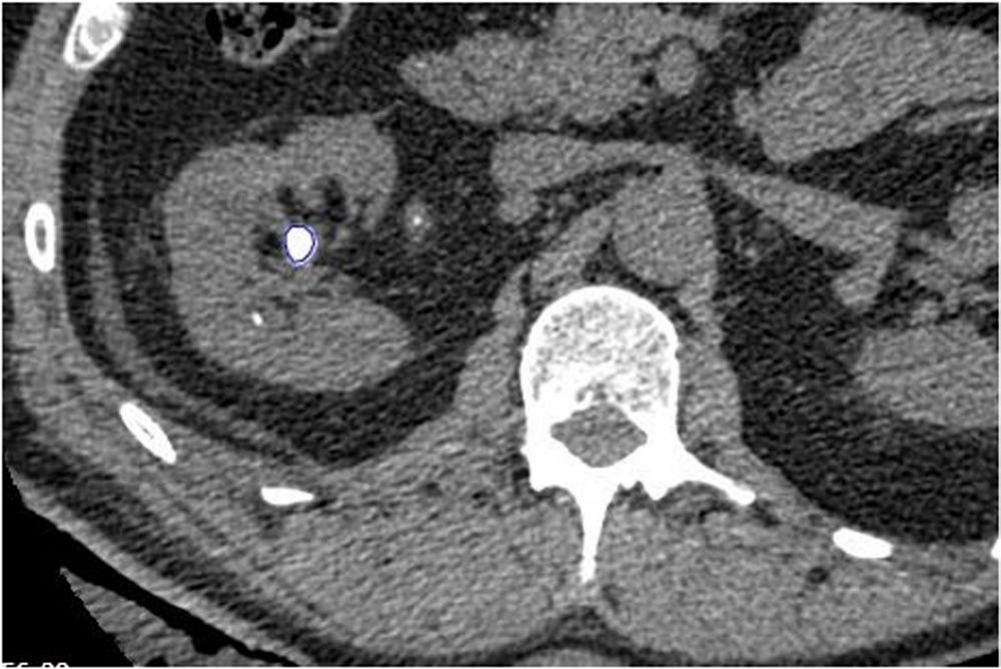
Example of a Region of Interest (ROI) automatically drawn (in blue) using CT textural analysis software (CTTA) to fit the largest cross-sectional area of the largest stone being treated by shockwave lithotripsy.
